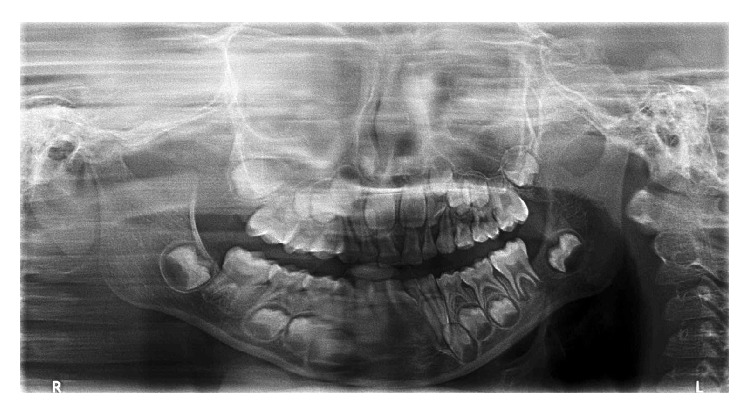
Orthopantomograph showing no bony involvement.
